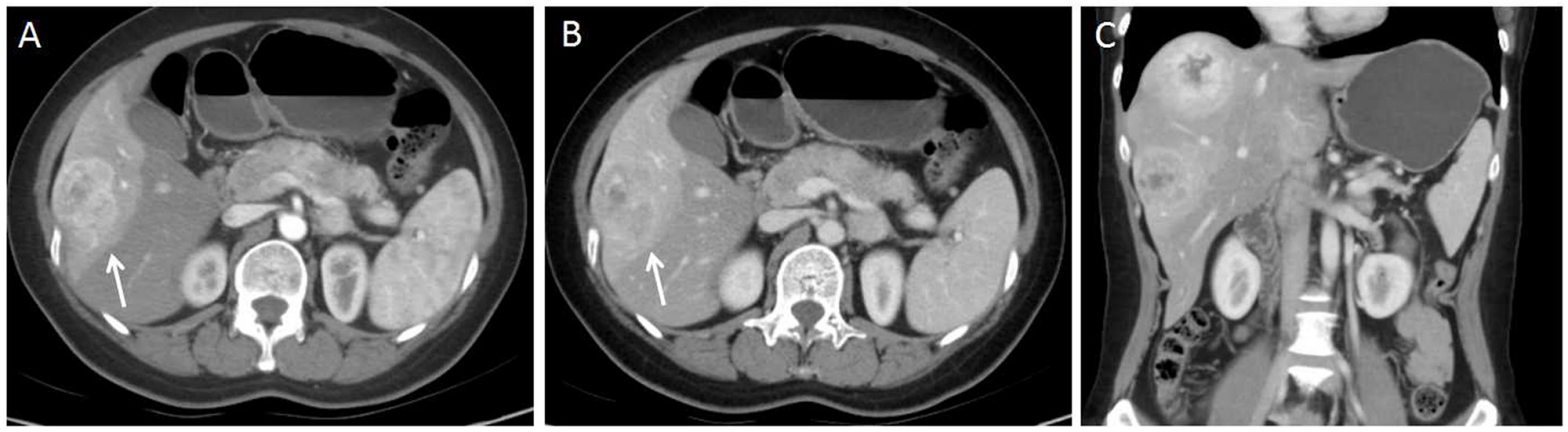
40-year-old woman with G2 pancreas NET (A) Axial CT image in the hepatic arterial phase shows a hyper enhanced liver metastasis in the right lobe of liver(arrow). (B) On portal venous phase, the lesion demonstrates washout enhancement pattern(arrow). (C) No regional or distal lymphadenopathy is present on coronal portal venous phase CT image.
